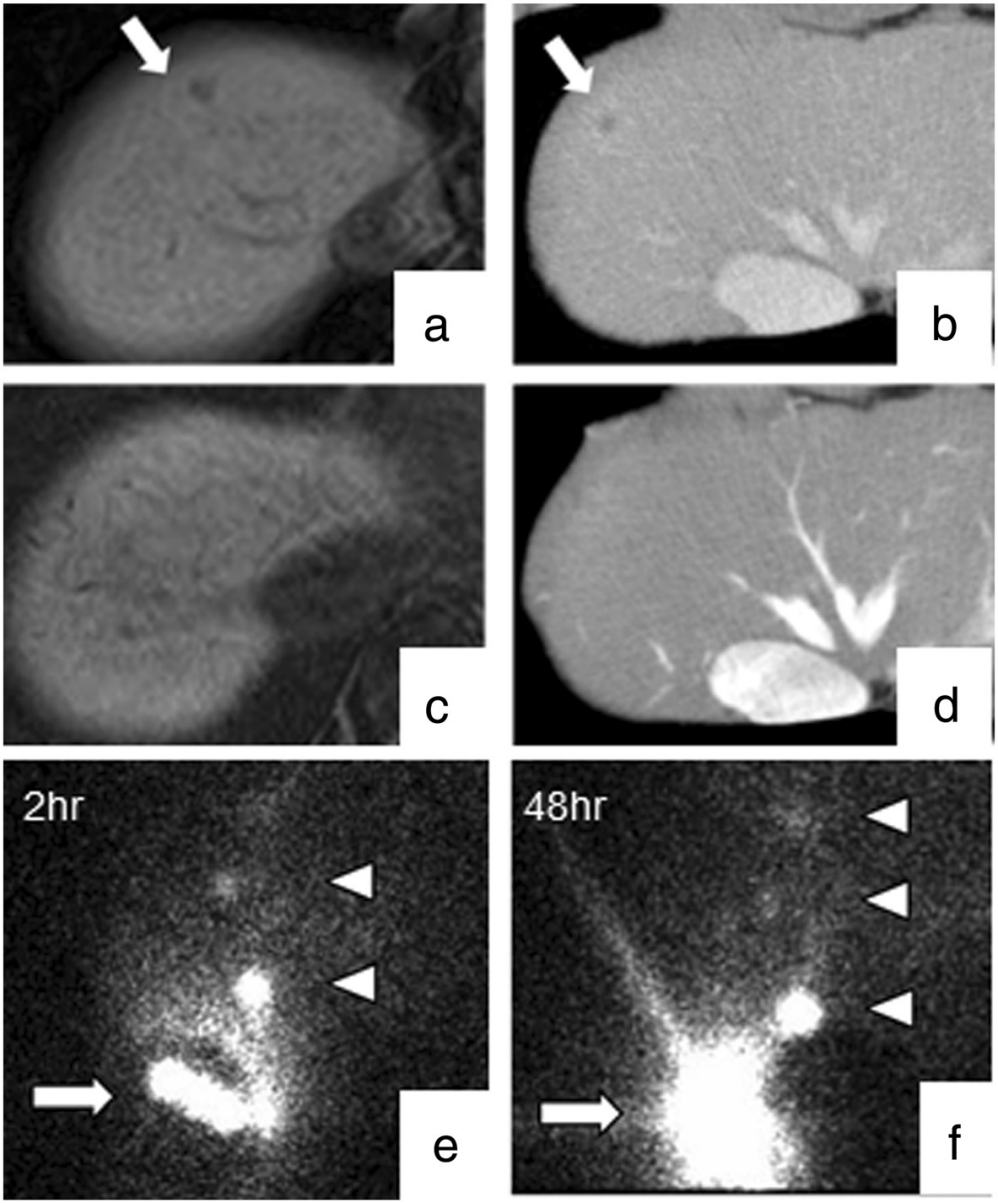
MRI (Gd-EOB-DTPA MRI, hepatobiliary phase) and CT scans of a patient with CR. Monitoring of in vivo migration of ^111^In-oxine labeled dendritic cells with scintigraphy. MRI and CT scans revealed a liver metastatic lesion (arrow) (a, b). After 3 cell transfers, the metastatic lesion disappeared completely (c, d). (a, c): MRI. (b,d): CT. Two hours after injection, DCs migrated from the injection site to inguinal lymph nodes (arrowhead) (e). Forty-eight hours after injection, that accumulation extended into distant lymph node, but still remained in the injection site (arrow) and inguinal lymph nodes (f).
